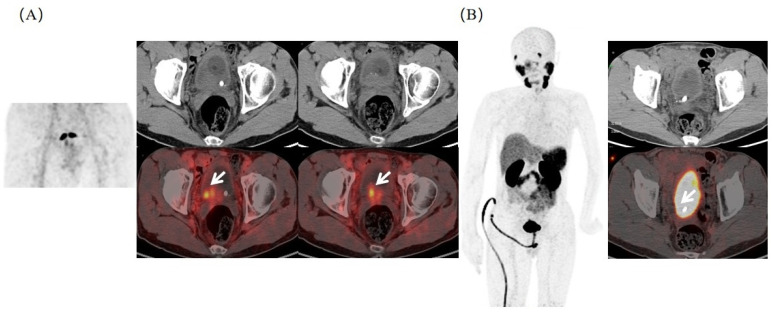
^68^Ga-PSMA-11 PET/CT in a 65-year-old PCa patient after endocrine therapy with elevated tPSA (42.18 ng/mL). (A) Focal increased ^68^Ga-PSMA-11 uptake (SUV_max_ = 22.7, T/BL ratio = 15.1) on the posterior wall of the bladder on fused axial PET/CT at 3 min P.I. (white arrow). (B) Corresponding bladder images at 45 min P.I., with markedly increased tracer accumulation. Differentiation of lesions and physiologic urethra activity was not possible.
